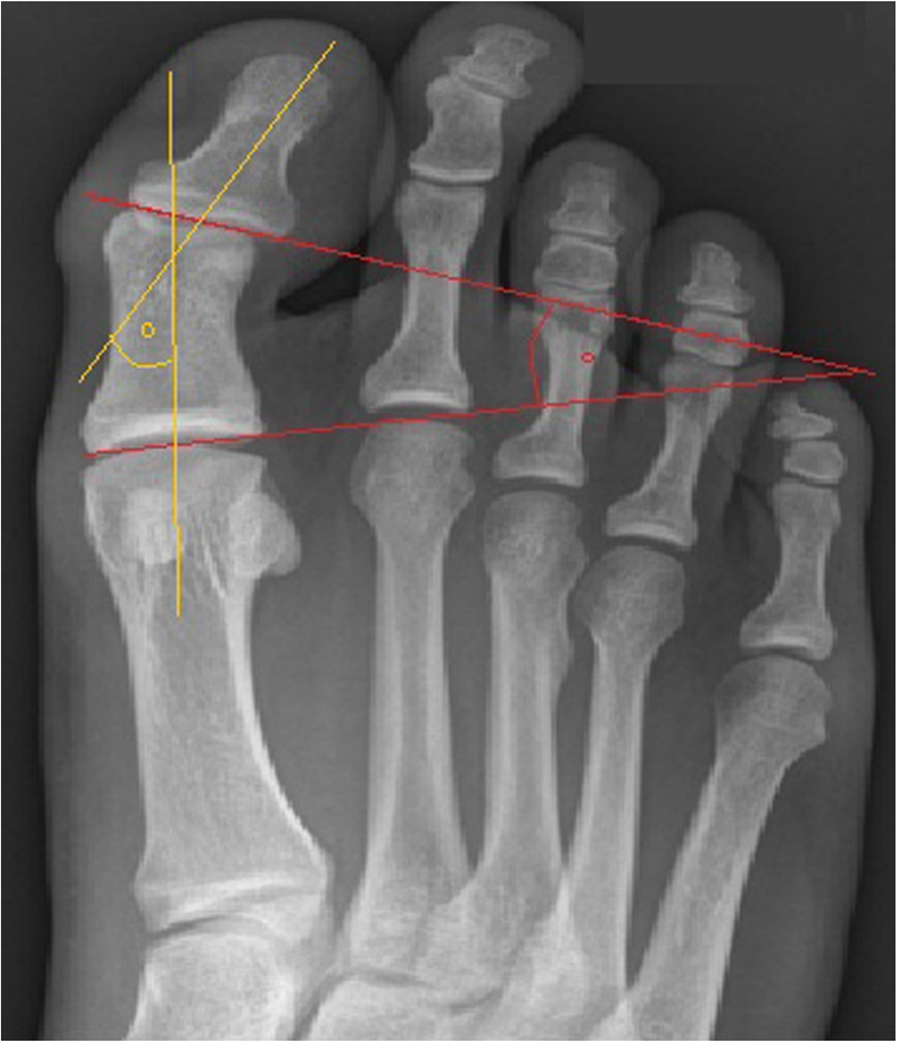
Dorsoplantar standing radiograph showing the projection of the PDPAA (red lines) and the HIA (yellow line)
